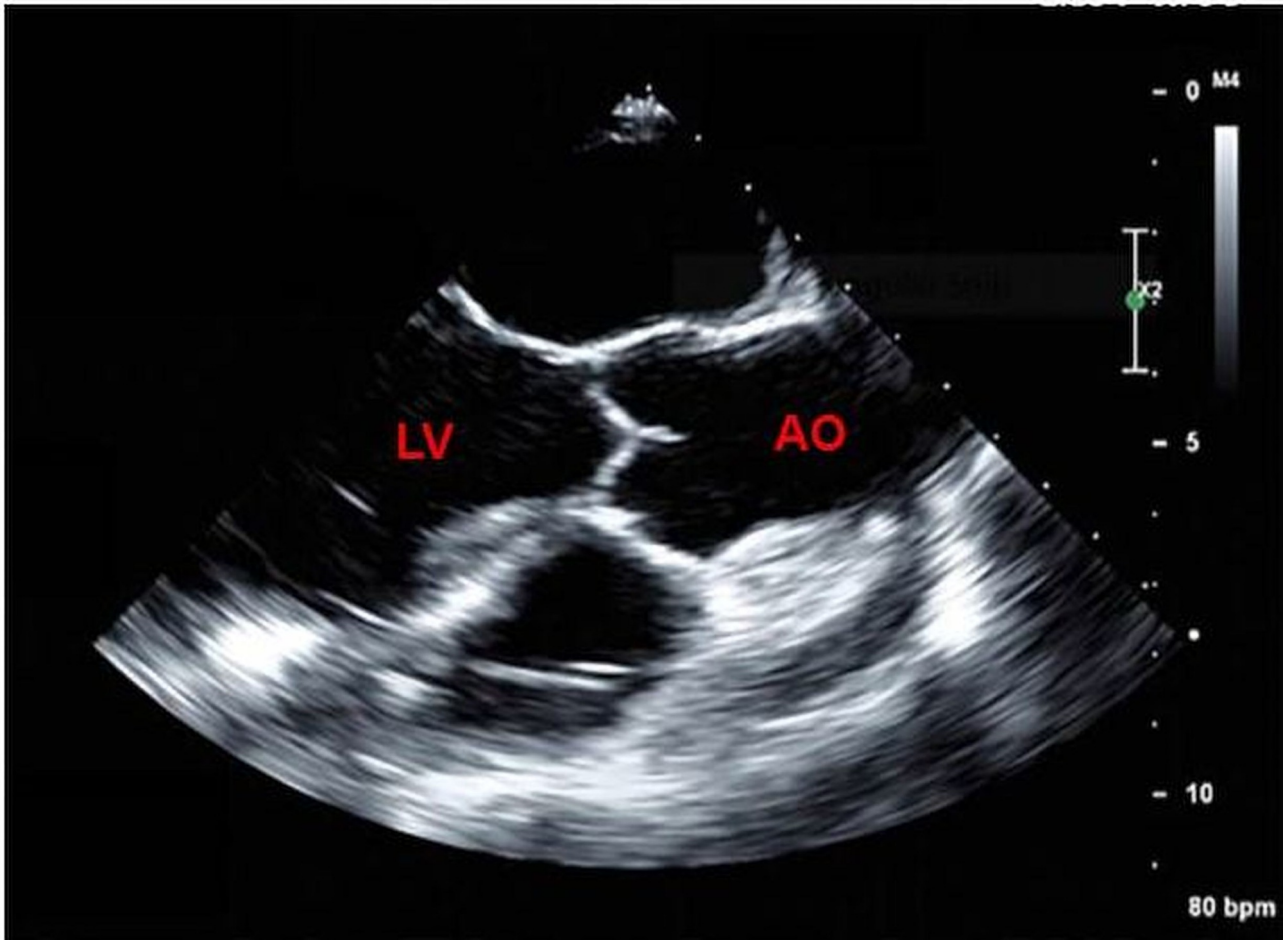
Postoperative transesophageal echocardiogram showed the absence of microbubbles LV: left ventricle; AO: aorta
